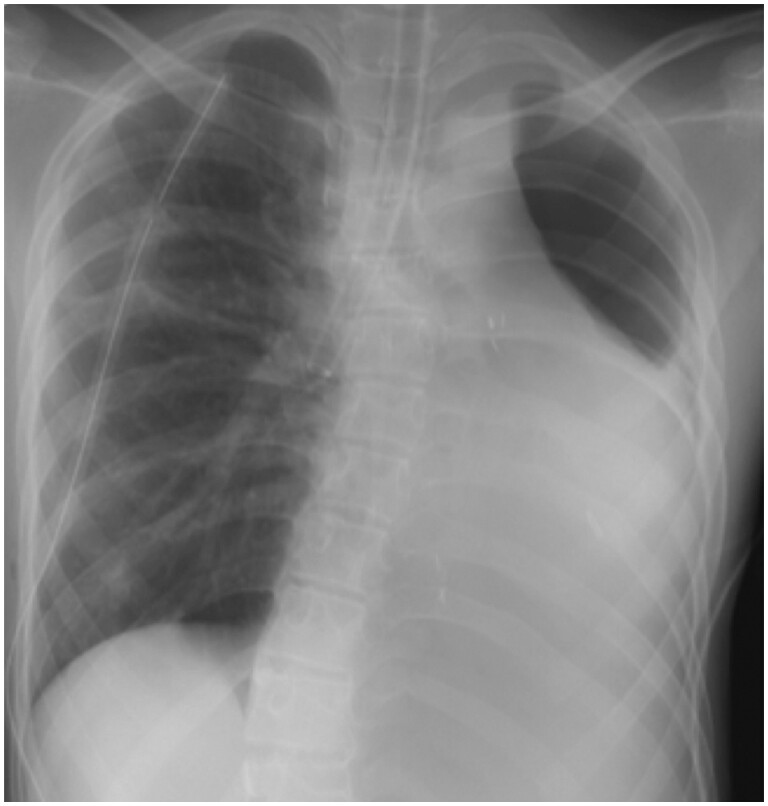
Chest radiography during surgery showed the tip of the left-sided double-lumen endobronchial tube in the right intermediate bronchus for selective lobar ventilation.
